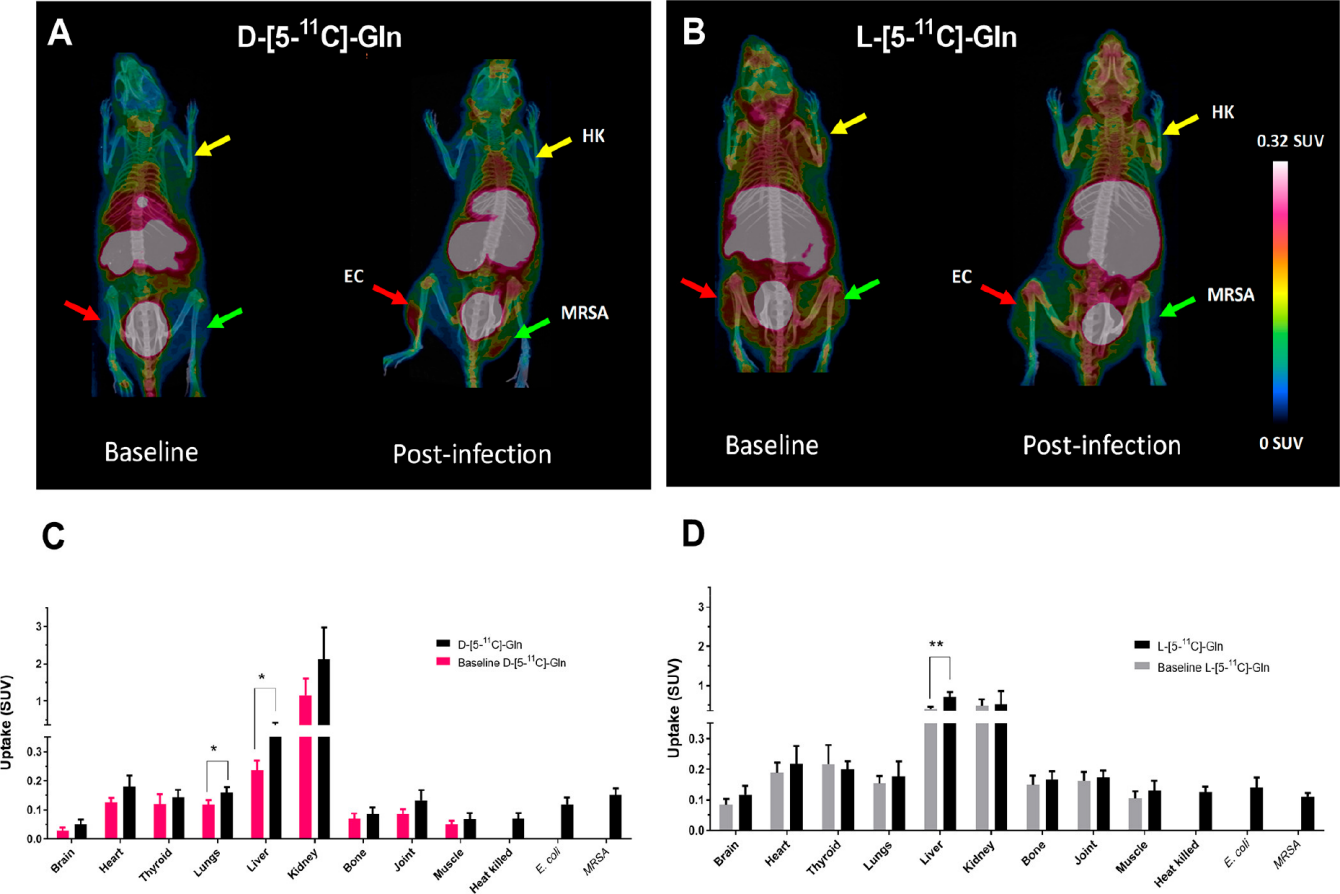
PET/CT scans with corresponding *ex vivo* biodistribution
